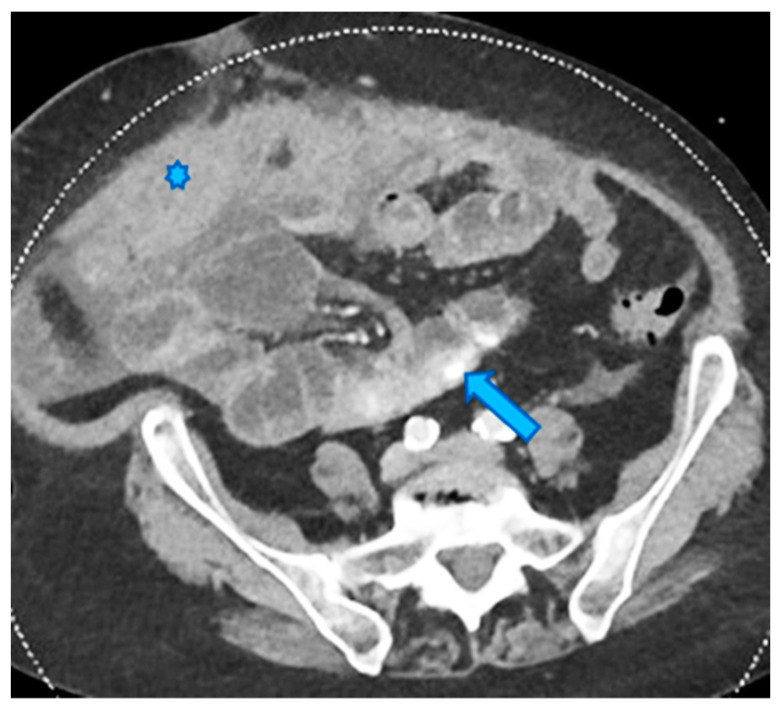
Axial CE-CT. PC from ovarian carcinoma: mesenteric seeding. Mesenteric involvement may occur as a combination of deposits involving both the mesentery and the bowel serosa, as in this case. Observe the clustered SB loops’ appearance. The calcified content of some of the deposits enhances their presence (arrow). Omental deposits (*).
